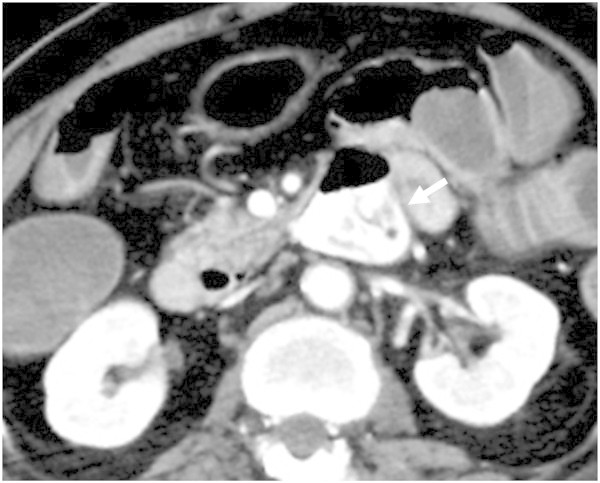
CT angiography via the venous approach depicts extravasation (arrow) of contrast medium in a large diverticulum in the ascending duodenum.
